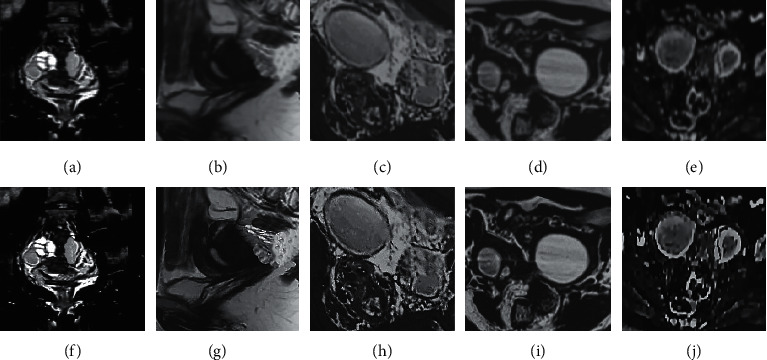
MRI images before and after processing by the artificial intelligence algorithm. (a–e) Traditional abdominal MRI images of coronal presaturated T2WI-FS, sagittal T2WI, sagittal presaturated T2WI-FS, sagittal T1WI, and sagittal DWI, respectively. (f–j) Abdominal MRI images processed by the improved artificial intelligence FCM algorithm of coronal presaturated T2WI-FS, sagittal T2WI, sagittal presaturated T2WI-FS, and sagittal T1WI, and sagittal DWI, respectively.
